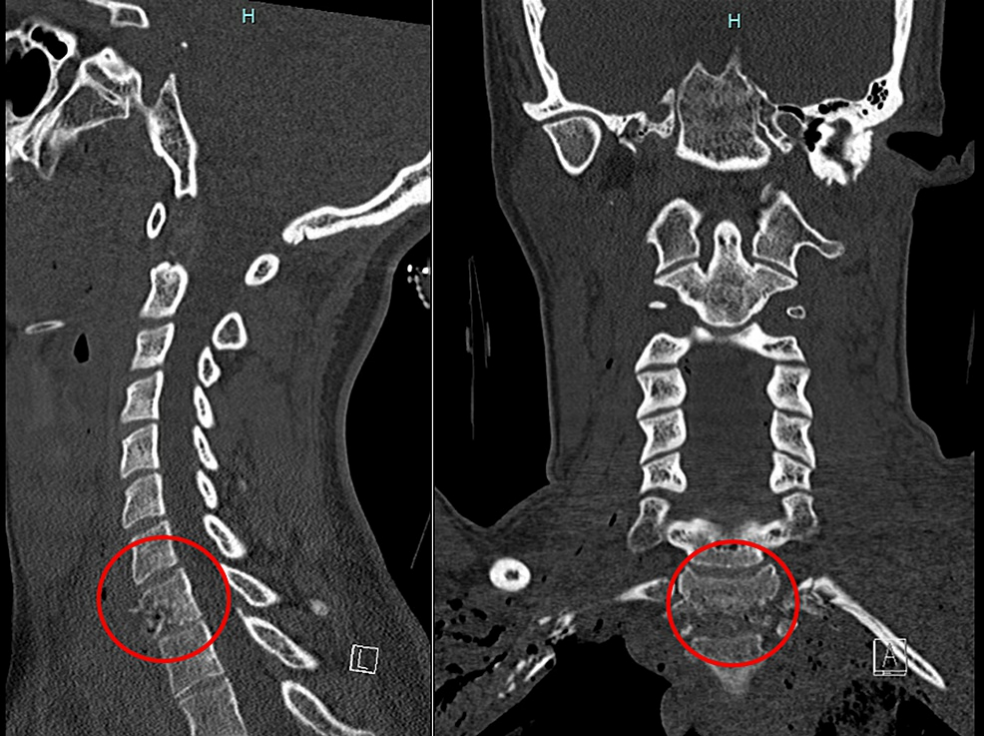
Sagittal and coronal CT of the cervical and thoracic spine showing a comminuted T1 fracture on the day of the trauma demonstrating an incomplete spinal cord injury (circled).
